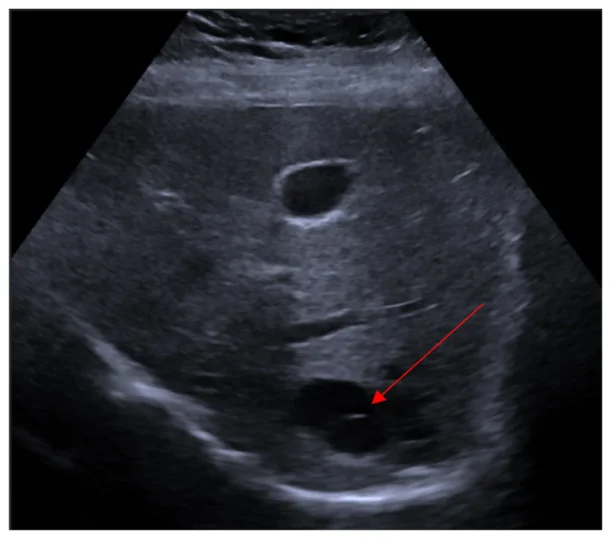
Abdominal ultrasound showing presence of a round cystic lesion in hepatic segment 6 with internal septation (red arrow).
